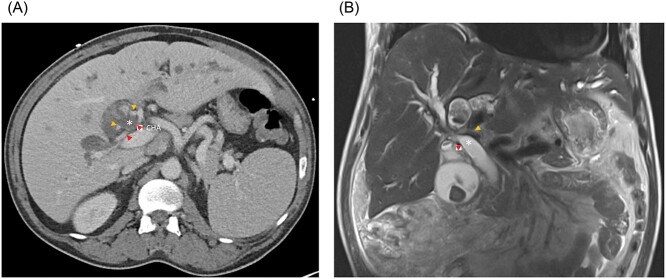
(A) Axial CT and (B) coronal MRI imaging showing the right anterior (orange arrowheads) and right posterior (red arrowheads) hepatic arteries encircling the common hepatic duct (white asterisk). CHA, common hepatic artery.
